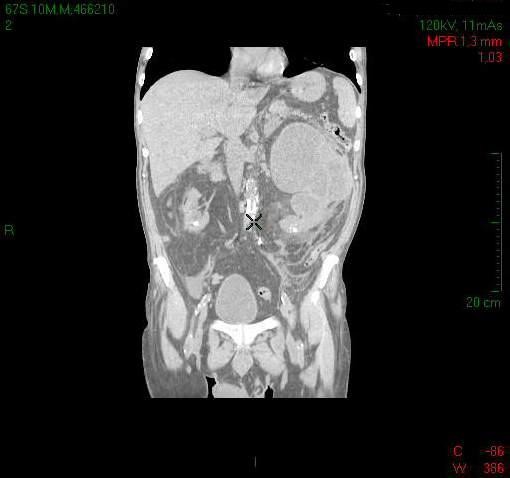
CT image depicting a grossly enlarged left kidney contained within the Gerota capsule.
